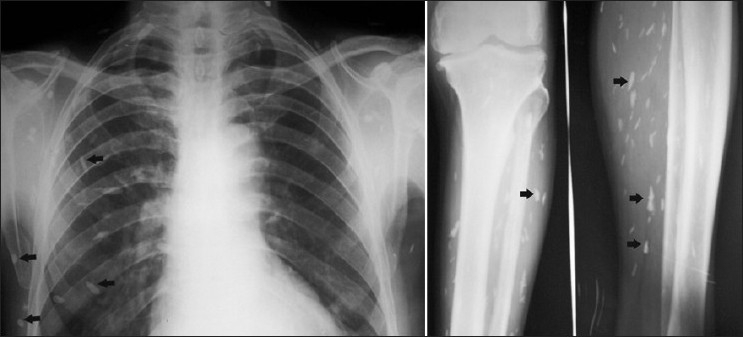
The chest radiograph showing multiple cigar-shaped calcifications in the subcutaneous plane (left arrows). The radiograph of the calf showing multiple cigar-shaped calcified lesions arranged in the subcutaneous plane and parallel to the muscle fibres in the intramuscular plane (centre and right arrows).
